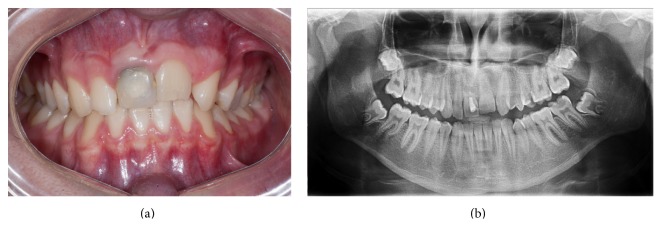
Six-year follow-up findings. (a) Intraoral photograph showing the discoloration of the maxillary right central incisor, infraocclusion of both central incisors, and midline shift. (b) Panoramic radiograph showing internal resorption in the maxillary right central incisor and ankylosis of the left central incisor.
